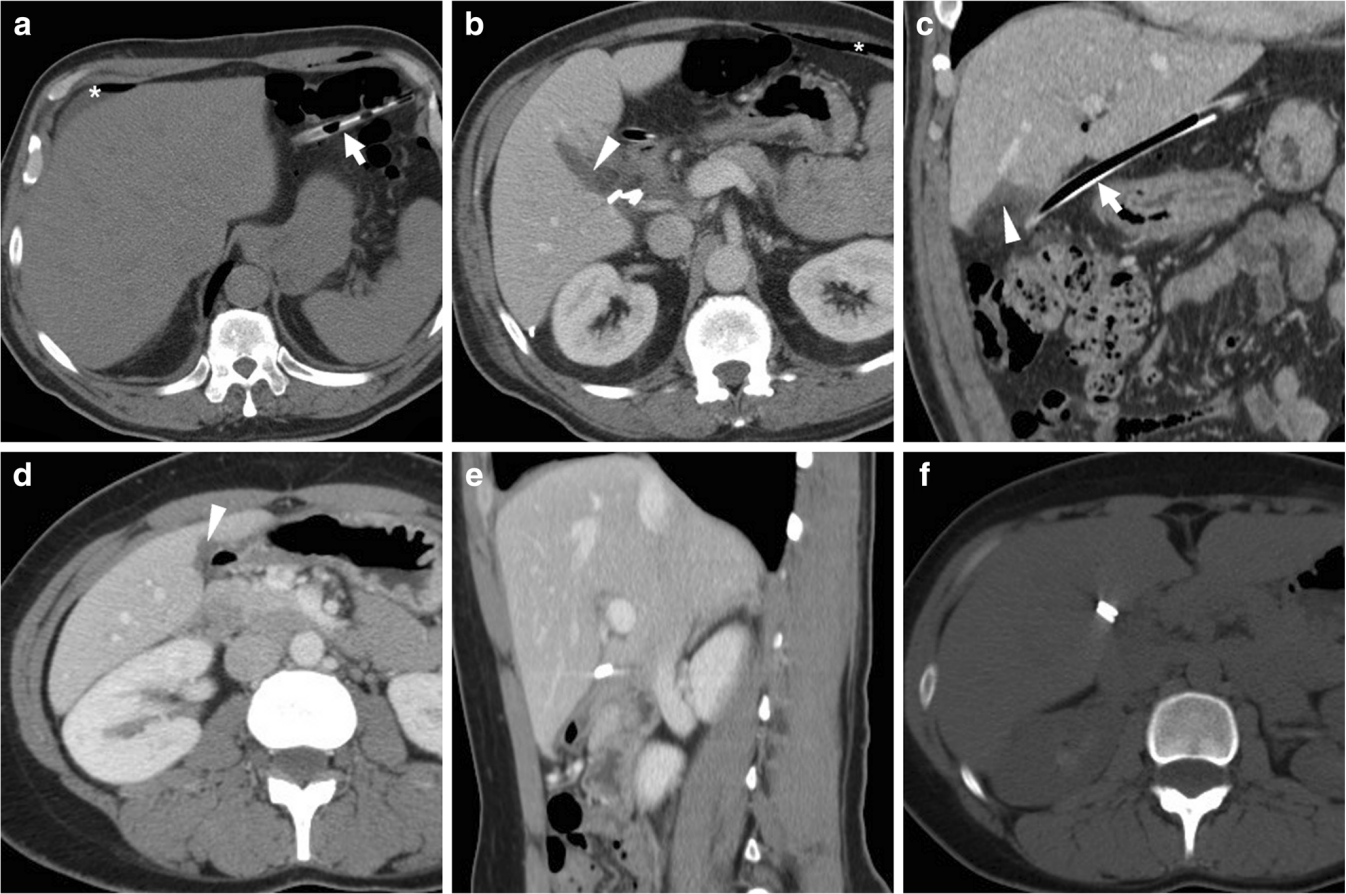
a–c Expected CT findings 48 h after uncomplicated open cholecystectomy, including minimal residual intraperitoneal air (* in a), drainage tube (*thick arrows*) in place and minimal fluid (*arrowheads*) in the operated gallbladder fossa adjacent to surgical clips. d–f Expected CT appearance shortly after laparoscopic cholecystectomy, with minimal fluid in the surgical bed (*arrowhead*); visualisation of metallic clips at the cystic duct and artery is improved by using bone window settings (f)
